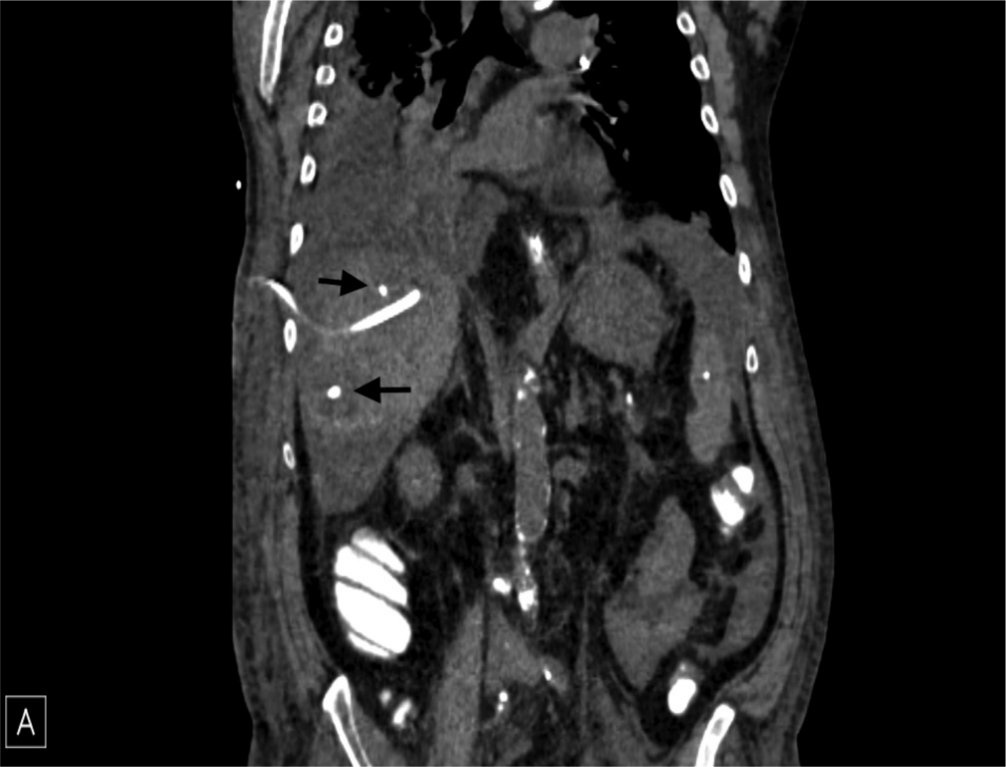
Computed tomography scan of the abdomen/pelvis without contrast showing intrahepatic drains near the hepatic abscesses.
